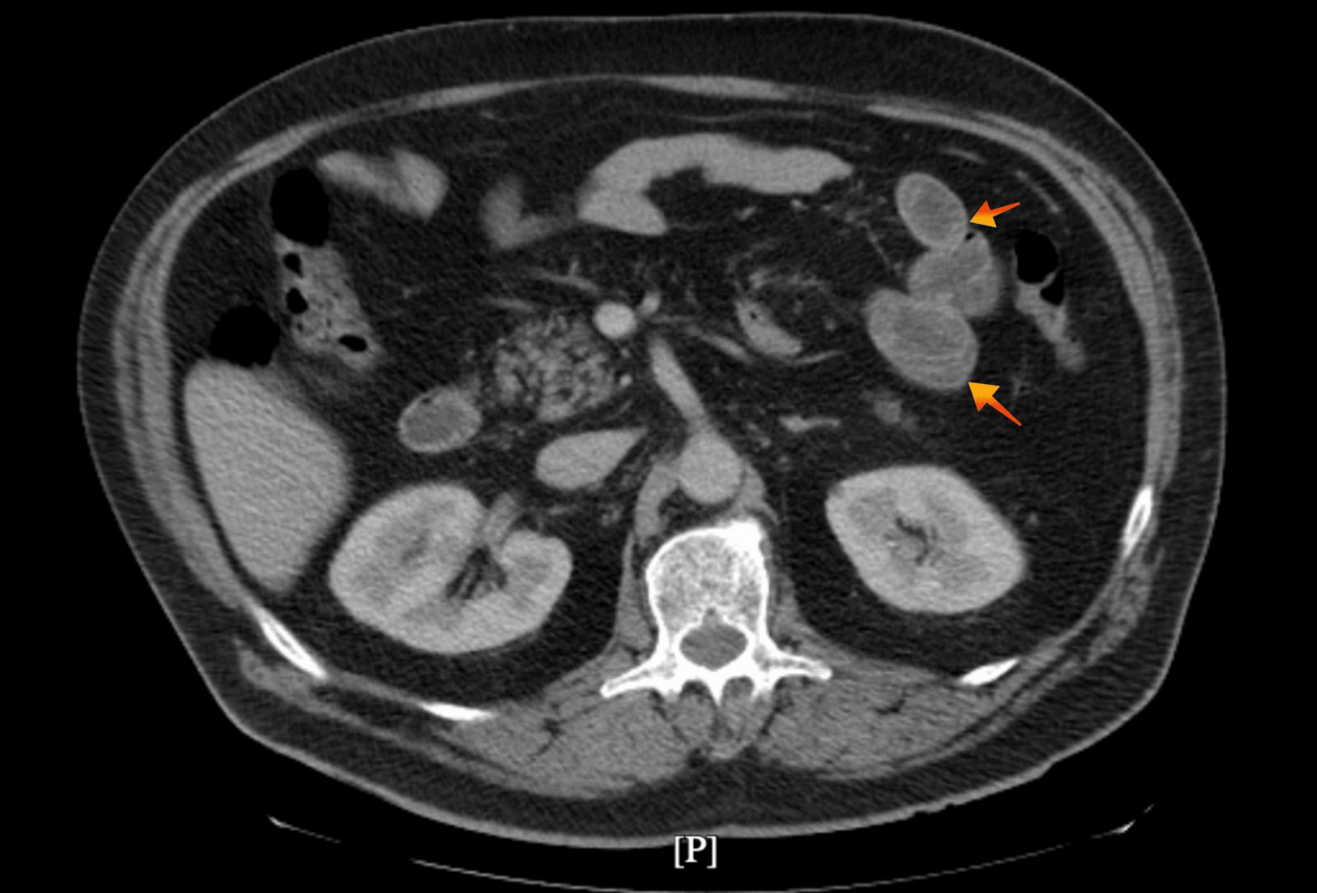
Computed tomography (CT) scan revealed features of acute enteritis. Bowel wall thickening and mucosal enhancement (arrows).
